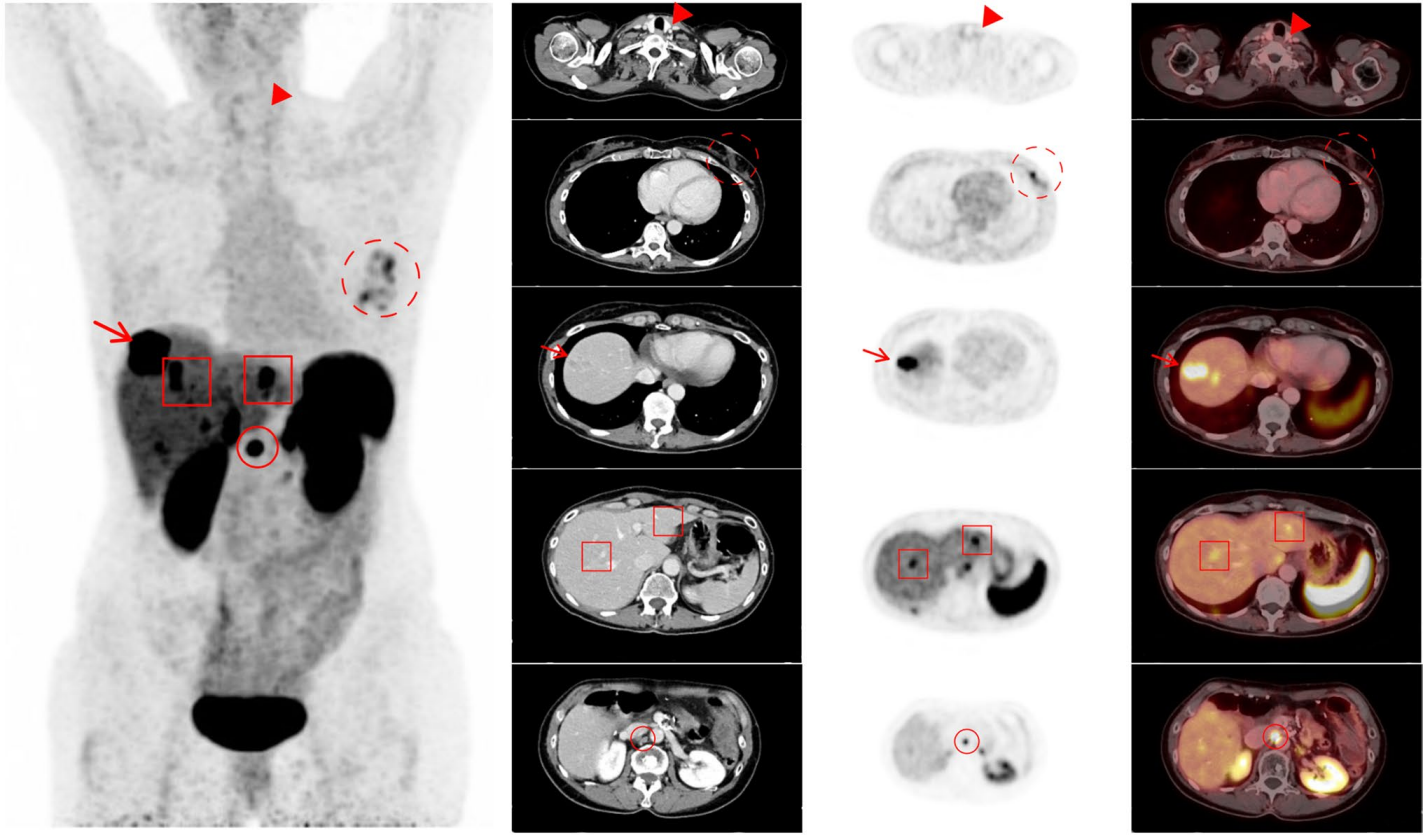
50-year-old woman with neuroendocrine tumor of the pancreas. The patient underwent contrast-enhanced diagnostic CT. The thyroid gland was defined as SSTR-RADS 1, with no abnormal tracer uptake. Axial CT, ^68^ Ga-DOTATOC PET, and fused PET/CT show a visible lesion with moderate tracer uptake (dashed circle) in the left breast compatible with fibroadenoma. SSTR-RADS 3C was assigned by all readers except one ER in one read. There is intense focal uptake in the liver dome (arrow) with corresponding finding on CT. This lesion was classified as SSTR-RADS-5 by all 4 readers. However, there are more lesions (red square) in segment II and VIII with no corresponding finding on CT (assigned SSTR-RADS 4 by only 2 readers in both reads). Intense uptake in a mesenterial lymph node can be noted (red circle). All readers identified the corresponding finding on CT, so this lesion was classified as SSTR-RADS-5 by all readers. All 4 readers except one ER in one read recommended PRRT according to the SSTR-RADS 1.0 criteria in this patient
